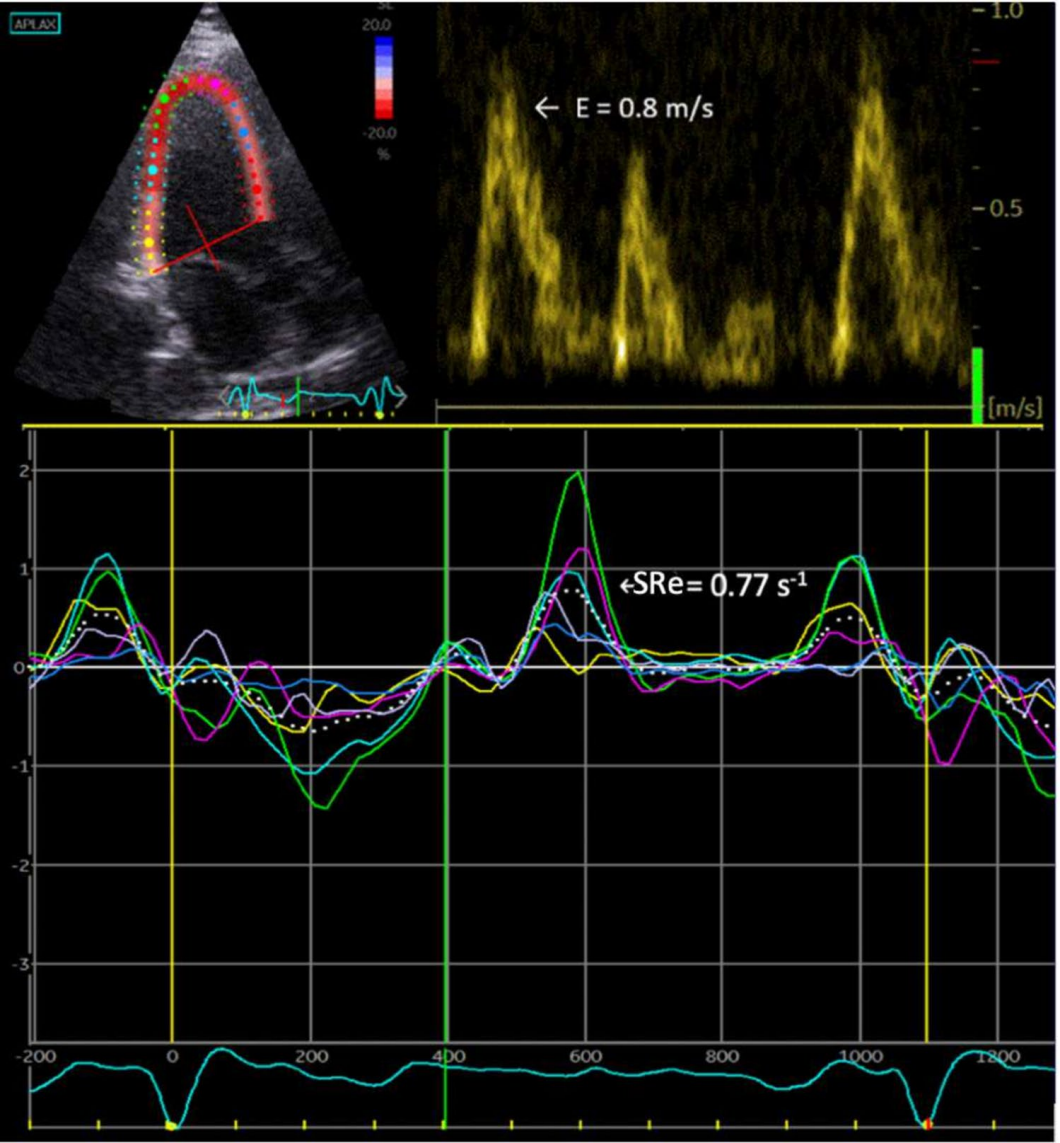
Example of E/SRe measurements. The figure shows an example of the measurements of early filling velocity (E) using pulsed-wave Doppler (top right), and early diastolic strain rate (SRe) from 2-dimensional speckle tracking analysis (top left and bottom). The white dotted line shows the global value
